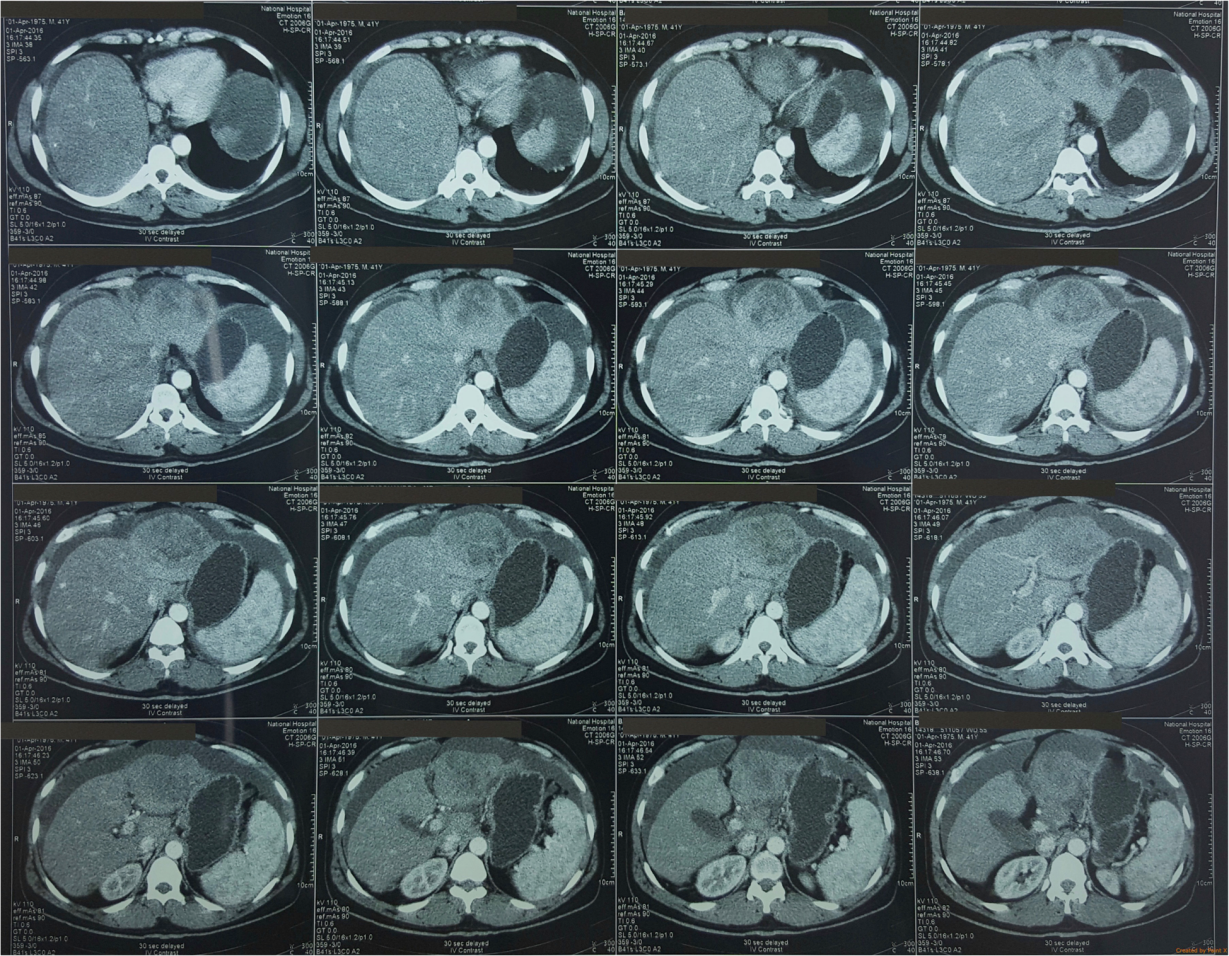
Liver lesion seen in 30 seconds, delayed film. Contrast-enhanced computed tomography of the abdomen
